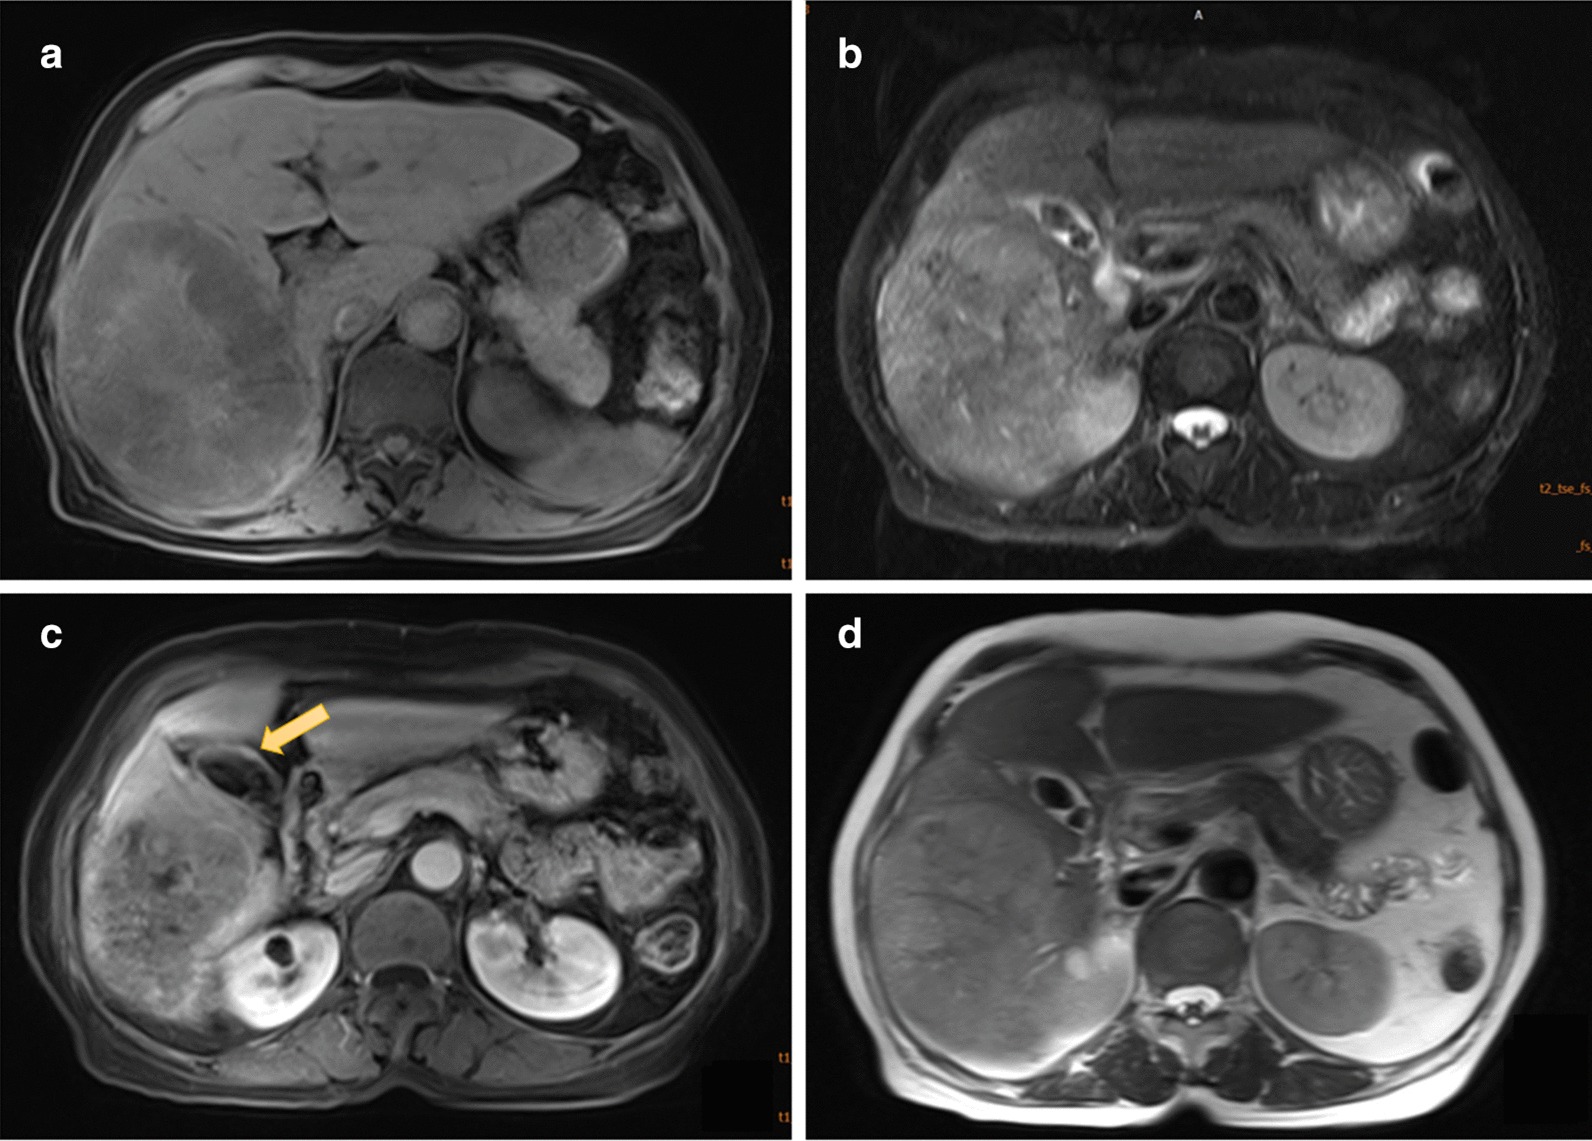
Abdominal MRI showed an 8.9 × 12.3 cm-sized mass, gallstones and slightly local nodular thickening in the gallbladder wall; a and c T1W images, b and d T2W images
